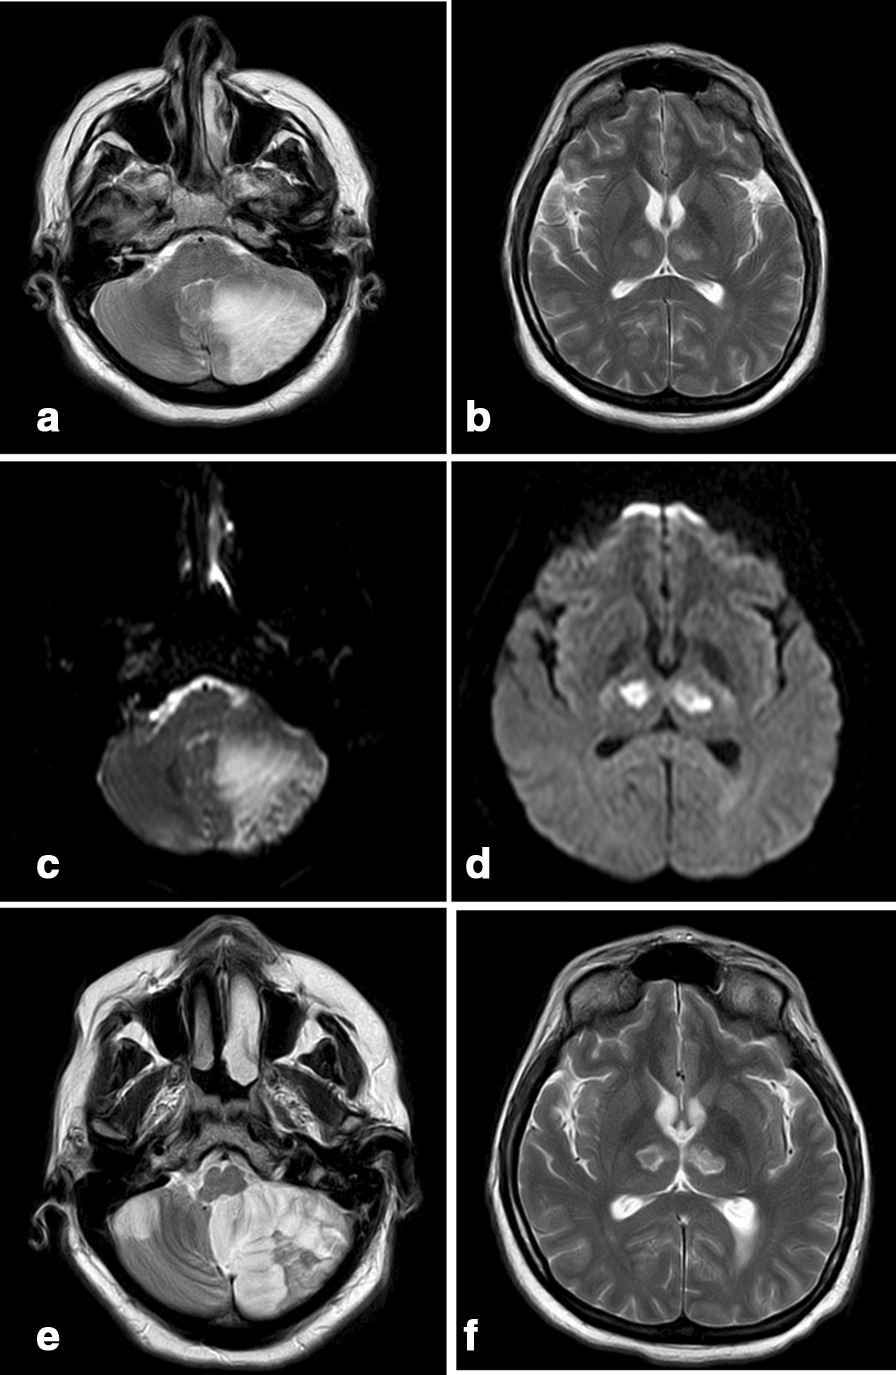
T2-weighted MRI showing a large infarct in the left cerebellar hemisphere (a) and rounded areas with high signal alteration in the medial thalami, corresponding to a bilateral paramedian thalamic infarct (b). Diffusion-weighted MRI demonstrating the left cerebellar (c) and bilateral thalamic infarcts (d). Second magnetic resonance imaging (MRI) 8 days later (e, f), T2-weighted MRI showing a new cerebellar infarct in the cerebellum’s right hemisphere (e)
